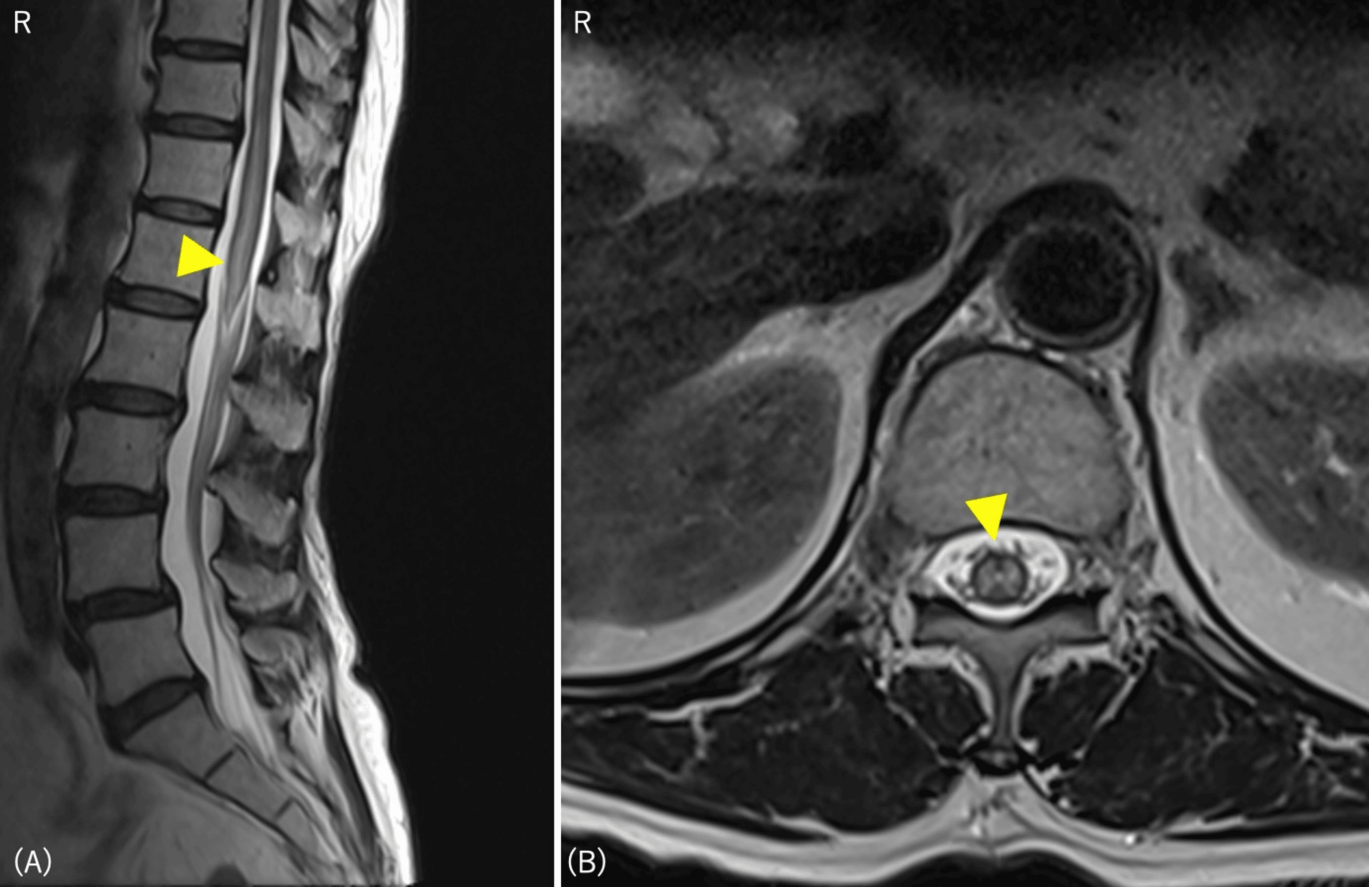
Spinal MRI scan (T2-weighted) on the first day of hospitalization (A) Sagittal section showing intramedullary hyperintensities at the level of the Th12-L1 vertebrae; (B) axial section: H-sign observed in the central gray matter.
